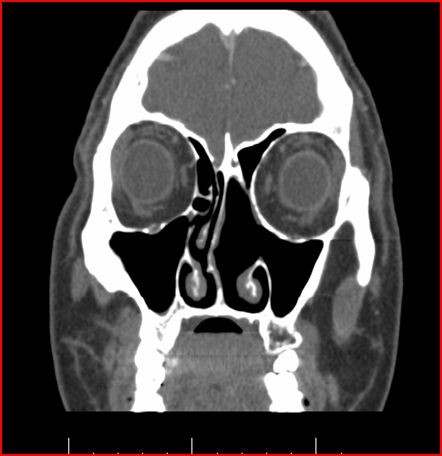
3 years postoperative CT Scan showing nasal cavity with no presence of tumor.
